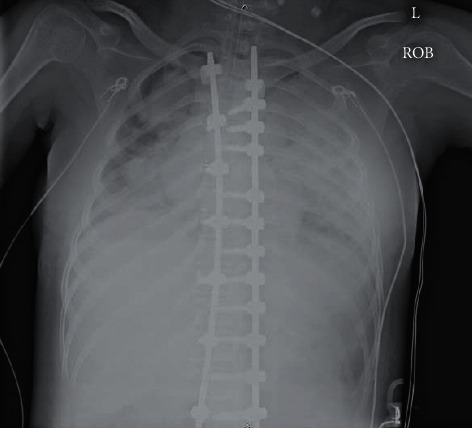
Initial chest X-ray demonstrated near-complete airspace consolidation on the left and patchy consolidations on the right.
